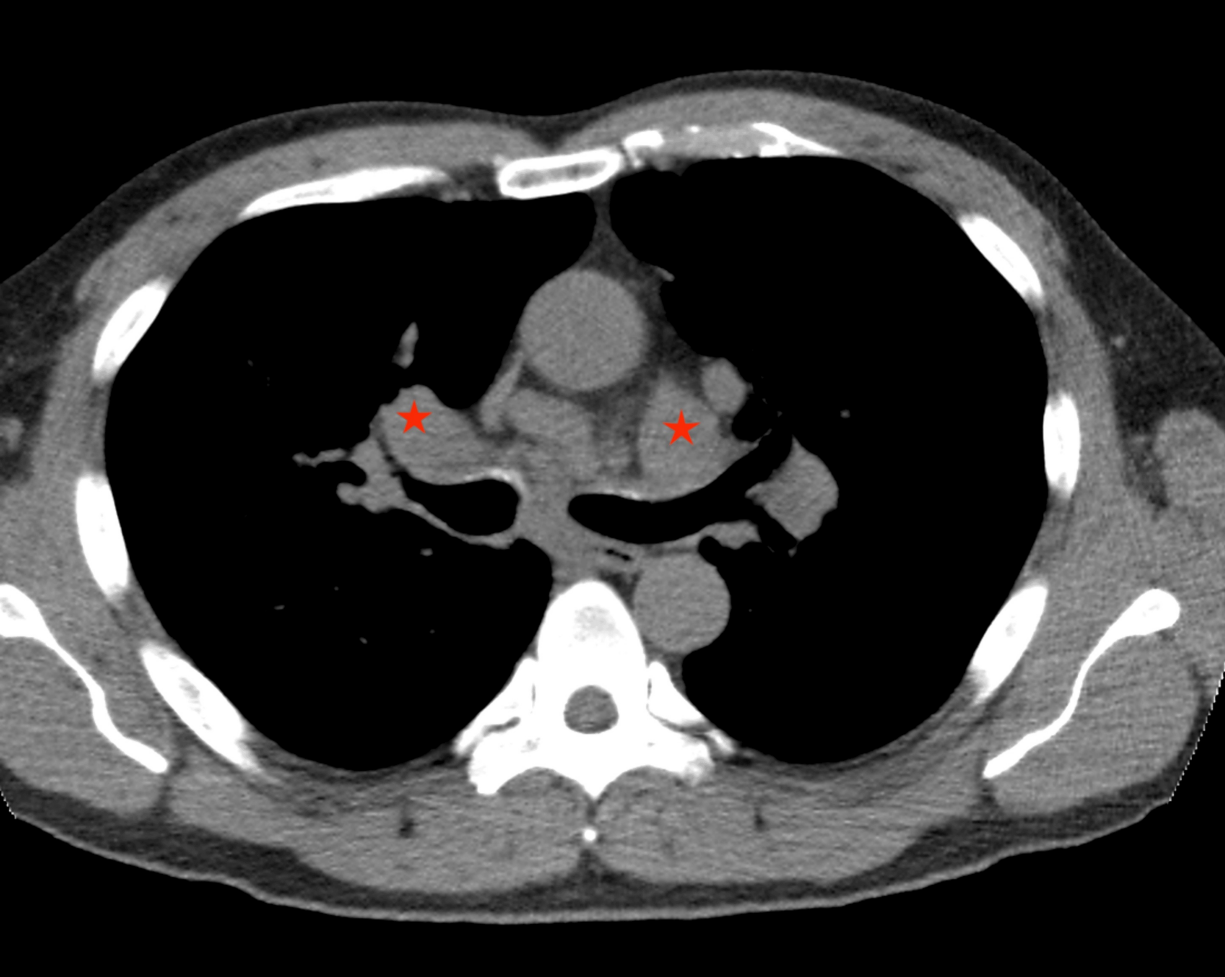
Axial CT chest showing bilateral hilar lymphadenopathy (red marks)
